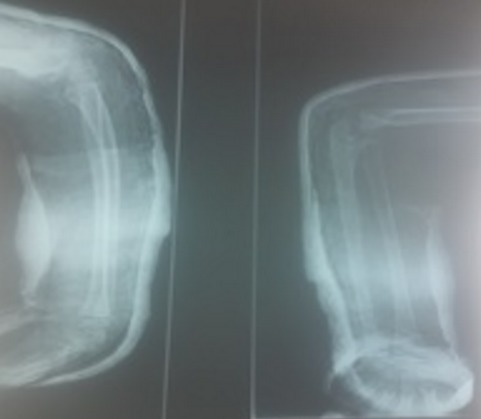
Radiographie de contrôle de l’avant-bras: réduction bonne
